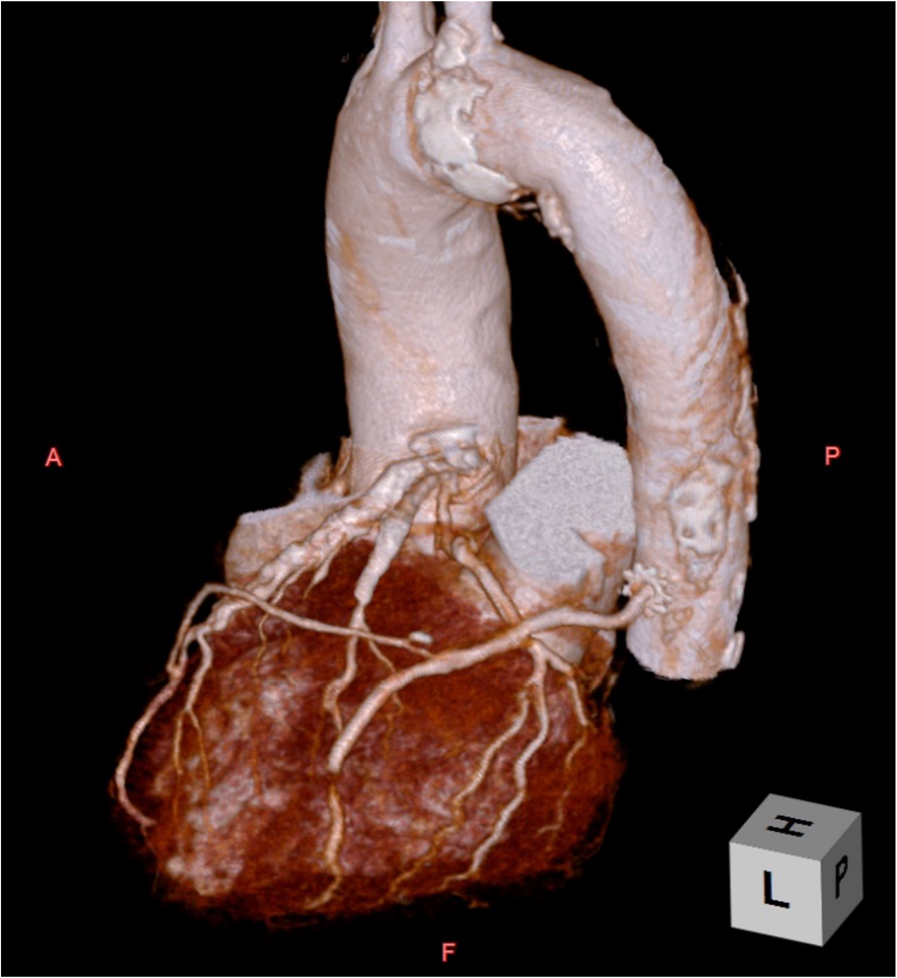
Postoperative three-dimensional coronary computed tomography angiography shows patent bypass grafts
